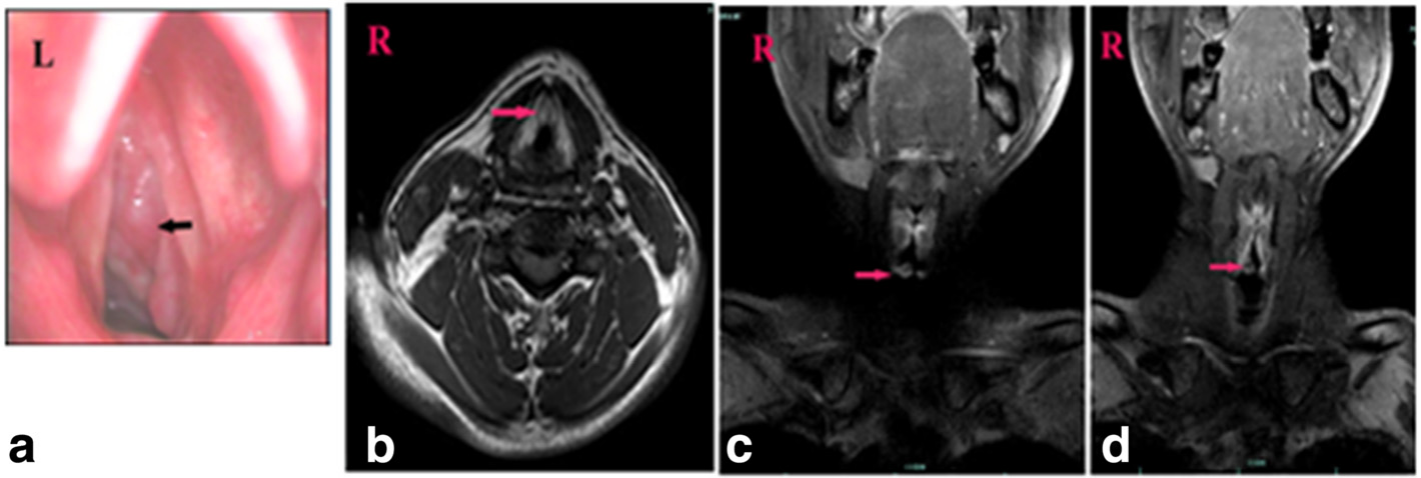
Laryngostroscopy and MRI of Case 7. a) Laryngostroscopy showed that there was a red smooth mass in the right subglottic area. An MRI scan revealed that there was a mass in the right subglottic area. b) TheT1-weighted signals, c) and the T2-weighted signals were hyperintense,d) and the contrast-enhanced T1-weighted MRI images show strong enhancement.
